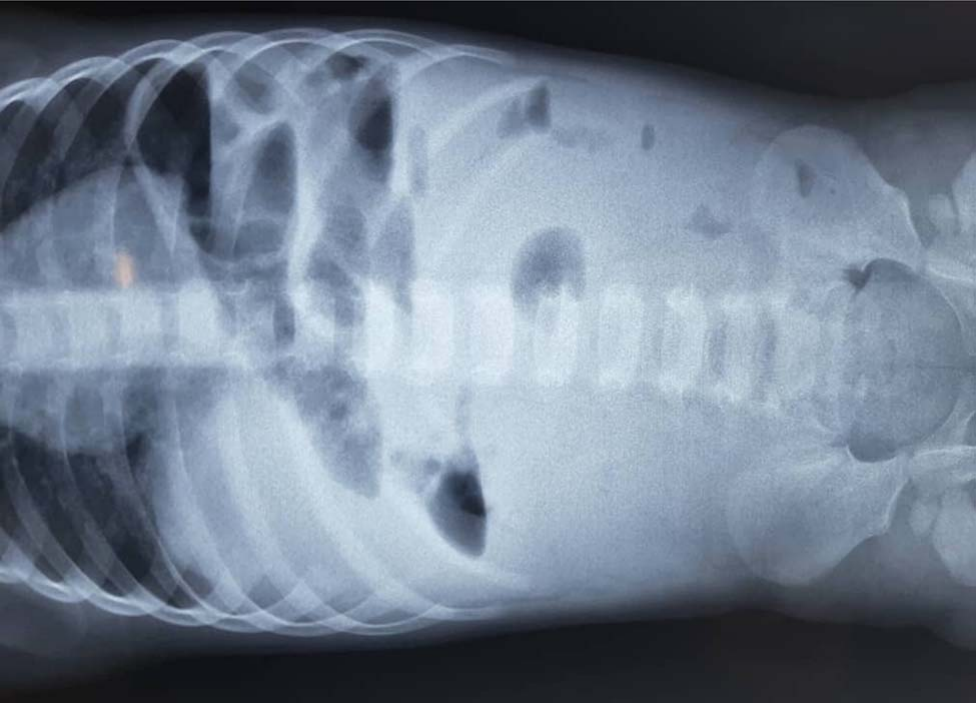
Abdominal X-ray after 6 h, which showed no change in appearance.
